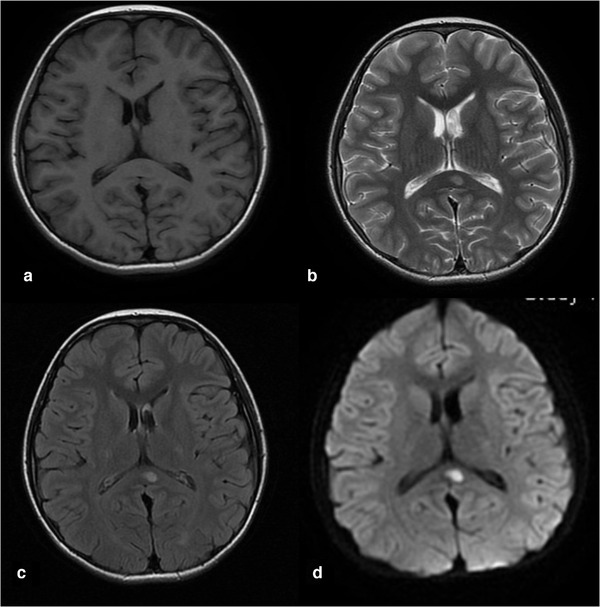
Cranial MRI revealed an isolated elliptic lesion in the splenium of the corpus callosum. The lesions were isointense to slightly hypointense on T1‐weighted images (a), homogeneously hyperintense on T2‐weighted images (b) and DWI (d), slightly hyperintense on T2‐FLARE (c)
